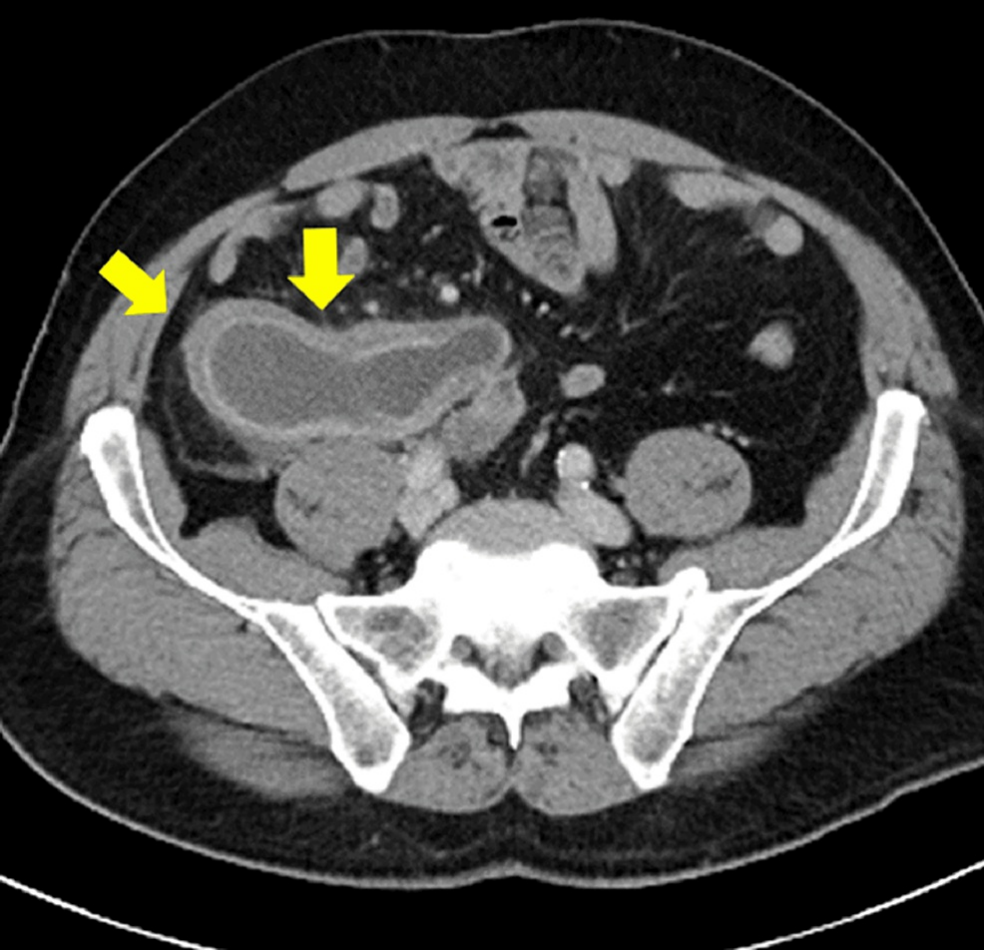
Contrast-enhanced computed tomography at the present time (axial image).
